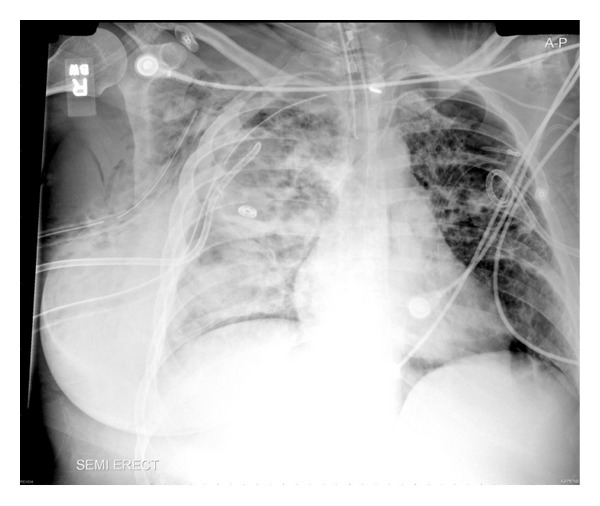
Chest X-ray of an ARDS victim who has developed multiple pneumothoraces secondary to a bronchopleural fistula.
